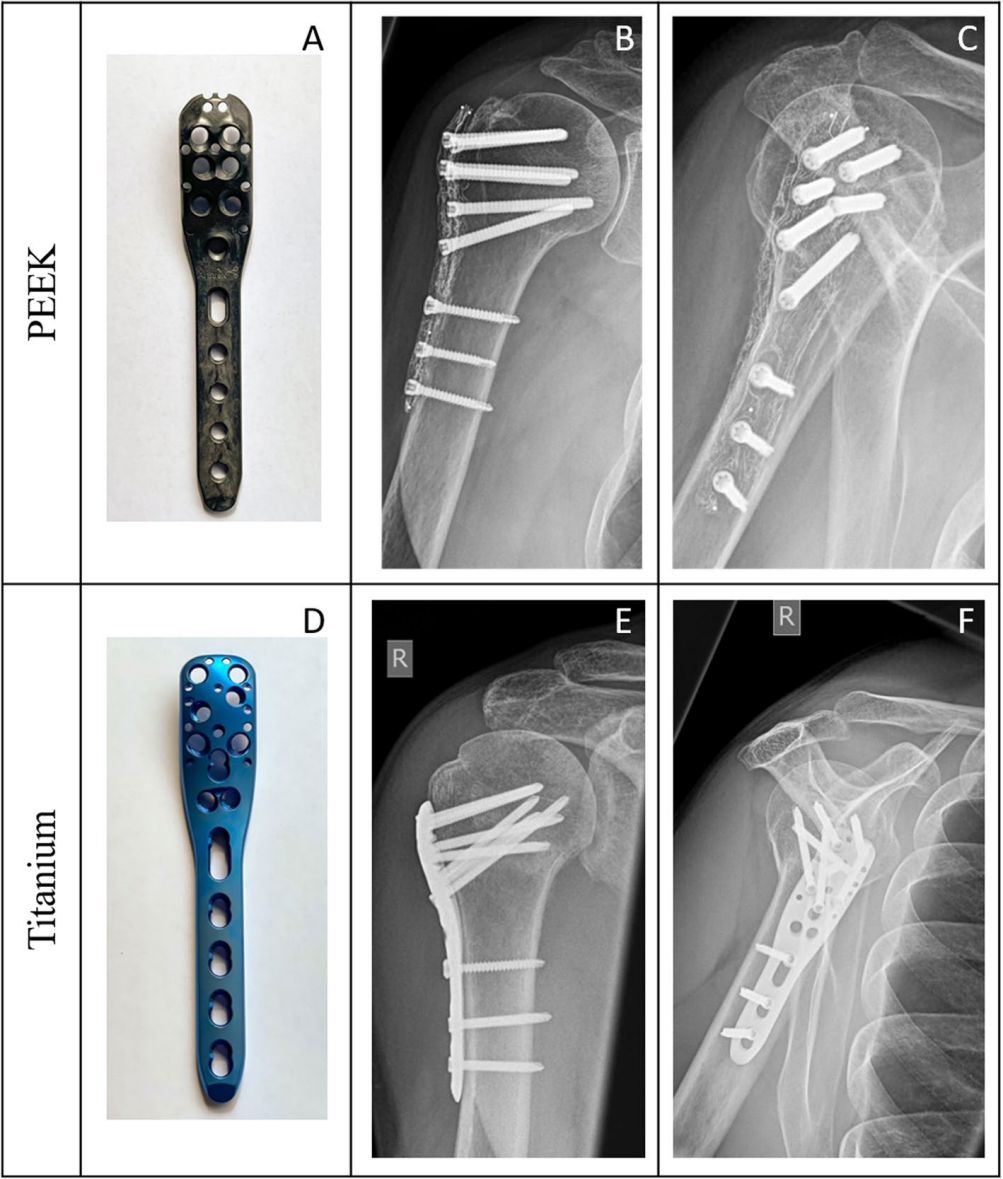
Photographs and radiographs (a.p. and lateral view) of a PEEK (A – C) and a titanium (D – F) plate. All radiographs show the last routine x-rays before implant removal. PEEK (A – C): female patient, 63 years at trauma, fracture classification according to AO 11-B1. Titanium (D – F): male patient, 45 years at trauma, fracture classification according to AO 11-B1
